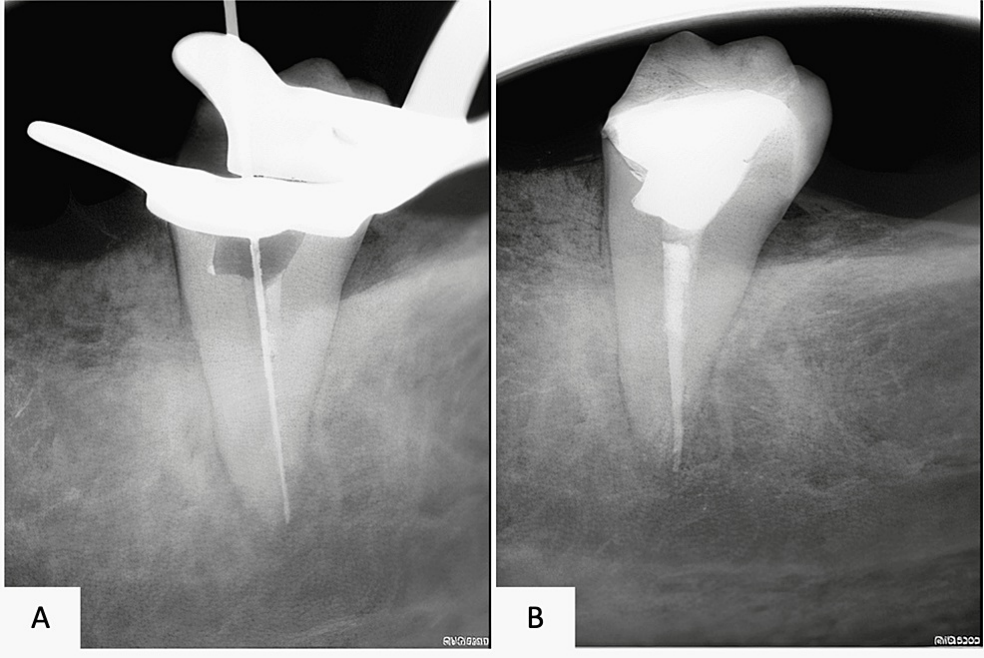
Intraoral periapical radiographs of tooth #37 A: Working length B: Final obturation
